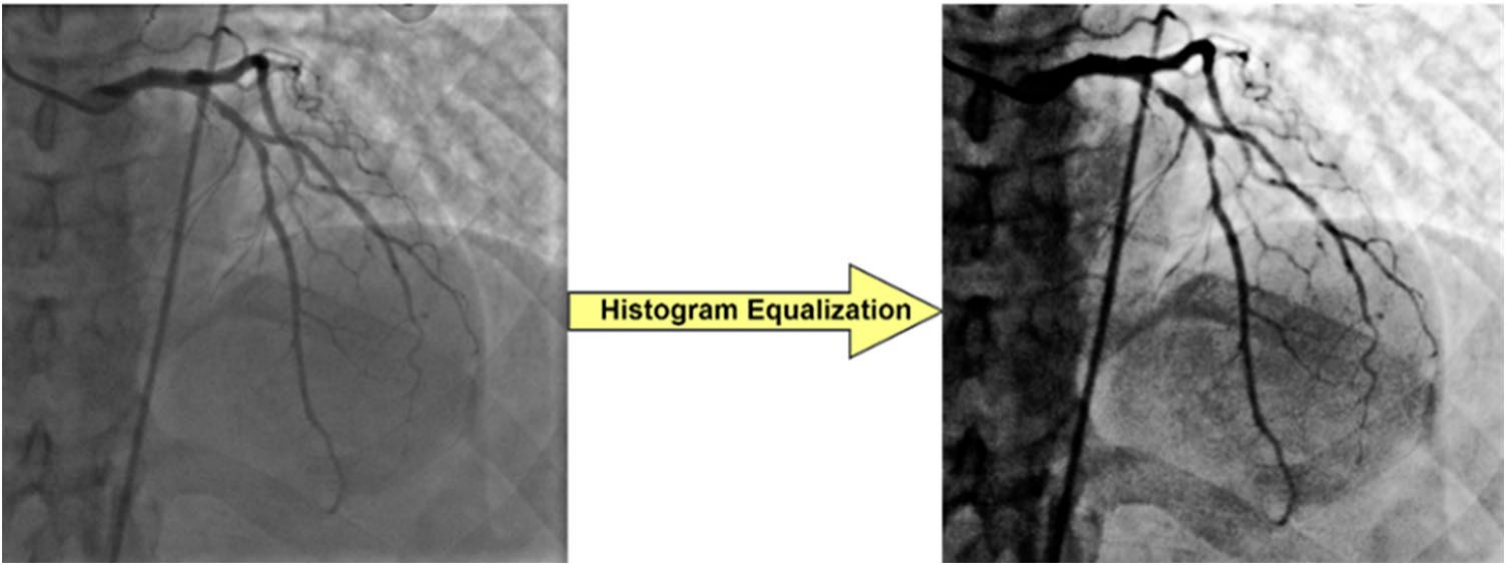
X-ray image before and after histogram equalization.
